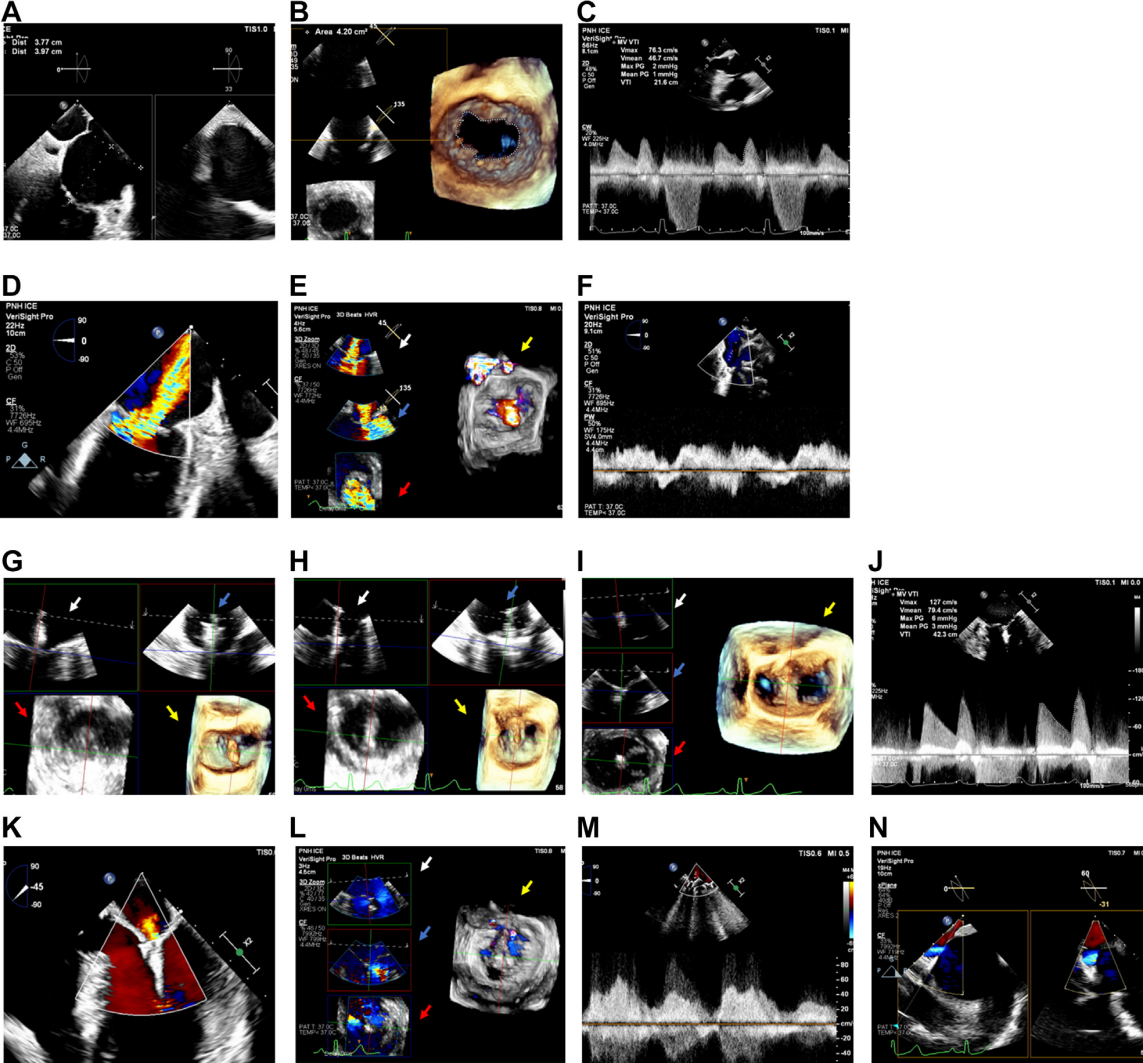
Mitral valve transcatheter edge-to-edge repair using 3-dimensionalintracardiacechocardiography (A) Transseptal puncture followed by detailed mitral valve (MV) evaluation using 3-dimensional (3D) intracardiac echocardiography, including (B) area by 3D planimetry, (C) mean gradient, as well as (D) mitral regurgitation assessment by 2-dimensional (2D) color imaging, (E) multiplanar reconstruction with color (bicommissural [white arrow], left ventricular outflow tract [blue arrow], en face 2D [red arrow], and en face 3D views [yellow arrow]), and (F) pulmonary vein Doppler analysis, revealing systolic blunting. Multiplanar imaging of the MitraClip in the (G) left atrium, (H) left ventricle, and (I) grasping the MV leaflets. (J) The final mean MV gradient. (K) 2D and (L) multiplanar views of MV leaflet insertion, with reduced mitral regurgitation. (M) Pulmonary vein Doppler, revealing systolic dominant flow. (N) 2D color X-plane image of residual interatrial shunt.
